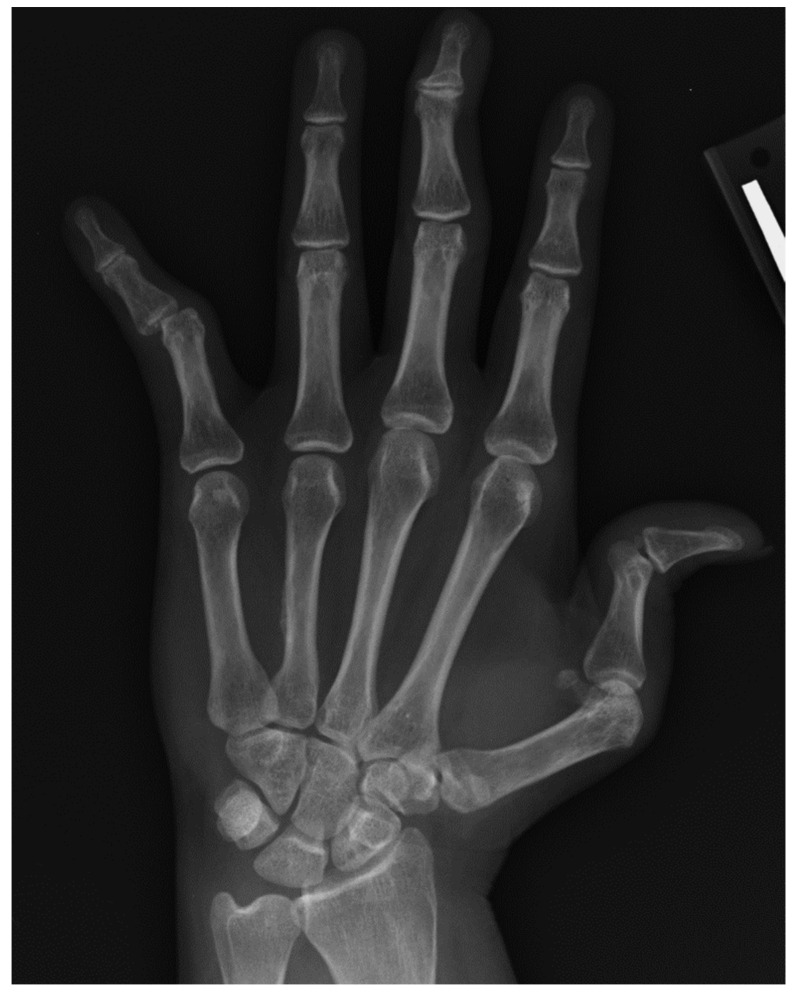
42-year-old female with SLE. Frontal hand radiograph with Boutonnière deformity of the thumb and ulnar subluxation of the fifth finger PIP joint. Note the absence of erosions.
